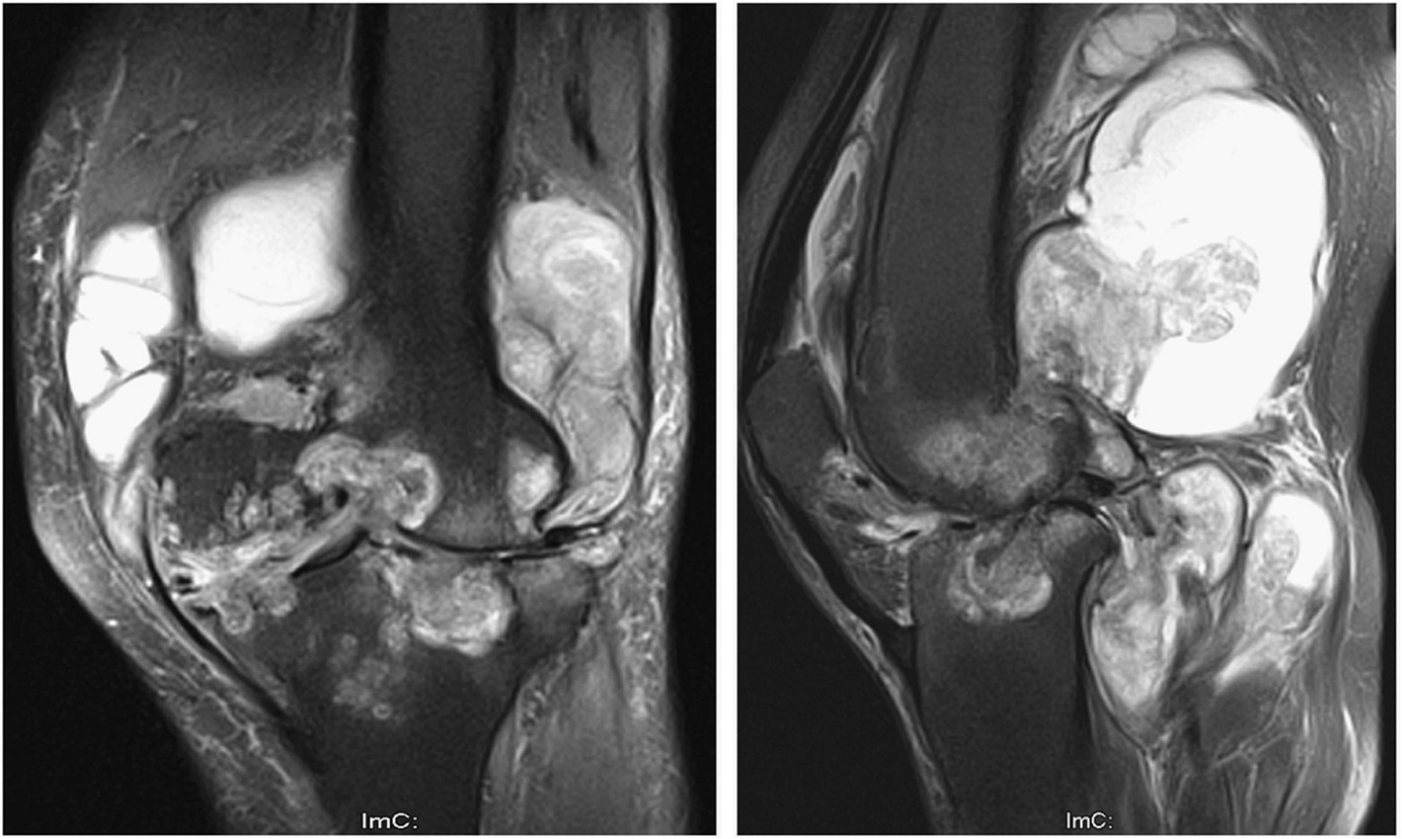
MRI (anteroposterior and lateral) showing severe joint destruction, obscure subcartilaginous bone plates, central and peripheral erosions, abscesses and articular cartilage damage
